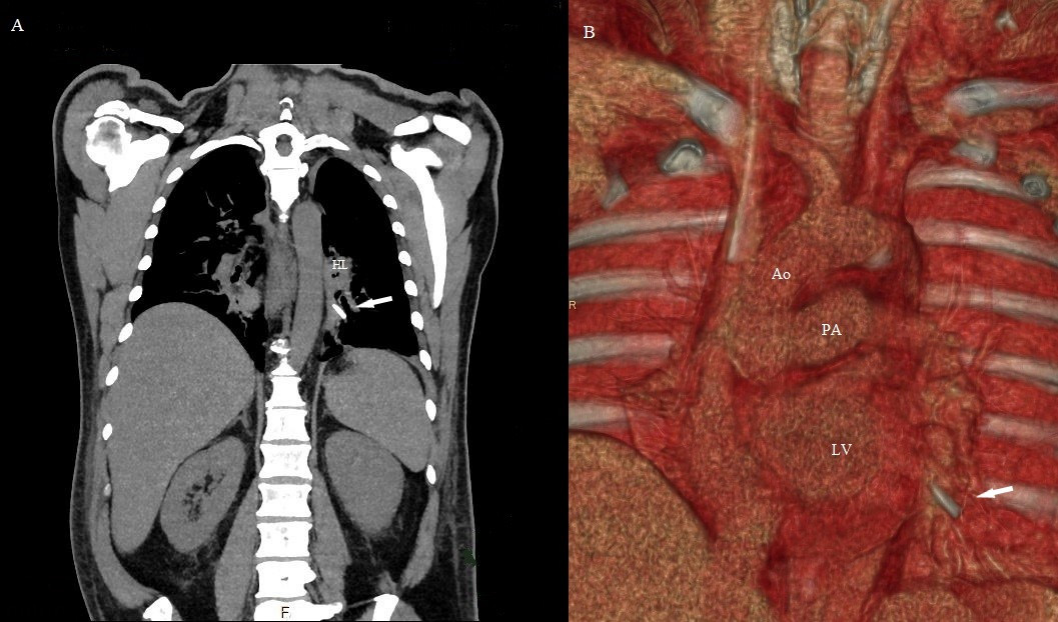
CT scan revealed a mechanical aortic valve hemidisc into the inferior left pulmonary vein. (A) Native computed tomography scan. (B) 3D volume rendering reconstruction of the chest. White arrow=artificial valve hemidisc. Ao=aorta; HL=hilum of the lung; LV=left ventricle; PA=pulmonary artery
